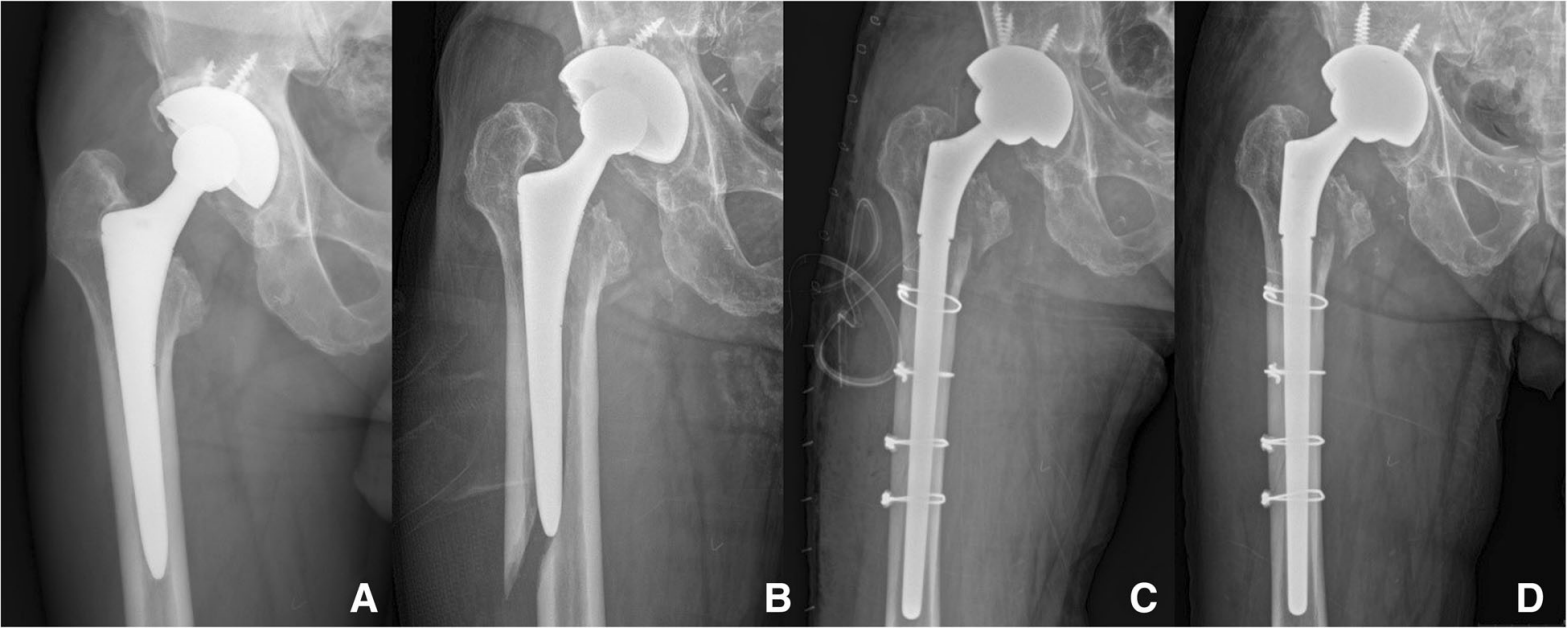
a Radiograph of the right hip joint 7 years after operation in a 71-old-year man who underwent left below knee amputation with total hip arthroplasty. The radiograph shows loosening of the components around the acetabulum and proximal femur. b A Vancouver type B2 fracture with stem loosening occurred after a fall at 8 years postoperatively. c Acetabular cup and femoral stem revision was performed using a long distal-fitting and modular-type stem with additional circular wiring. d At 24 months after revision surgery, the radiograph showed bony union and stable stem fixation
